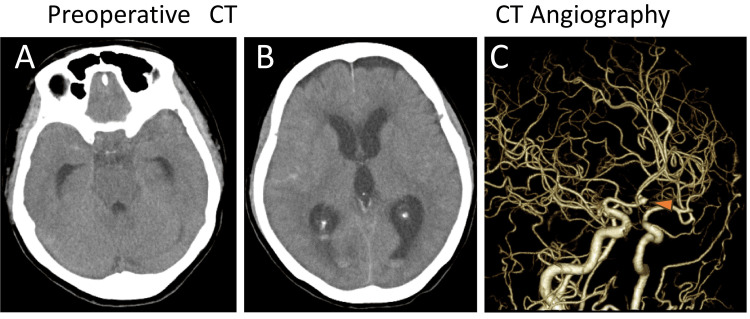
Preoperative images A CT scan confirmed SAH (A and B). CT angiography revealed a 5-millimeter ruptured aneurysm at the anterior communicating artery (orange arrowhead in C) CT: computed tomography
